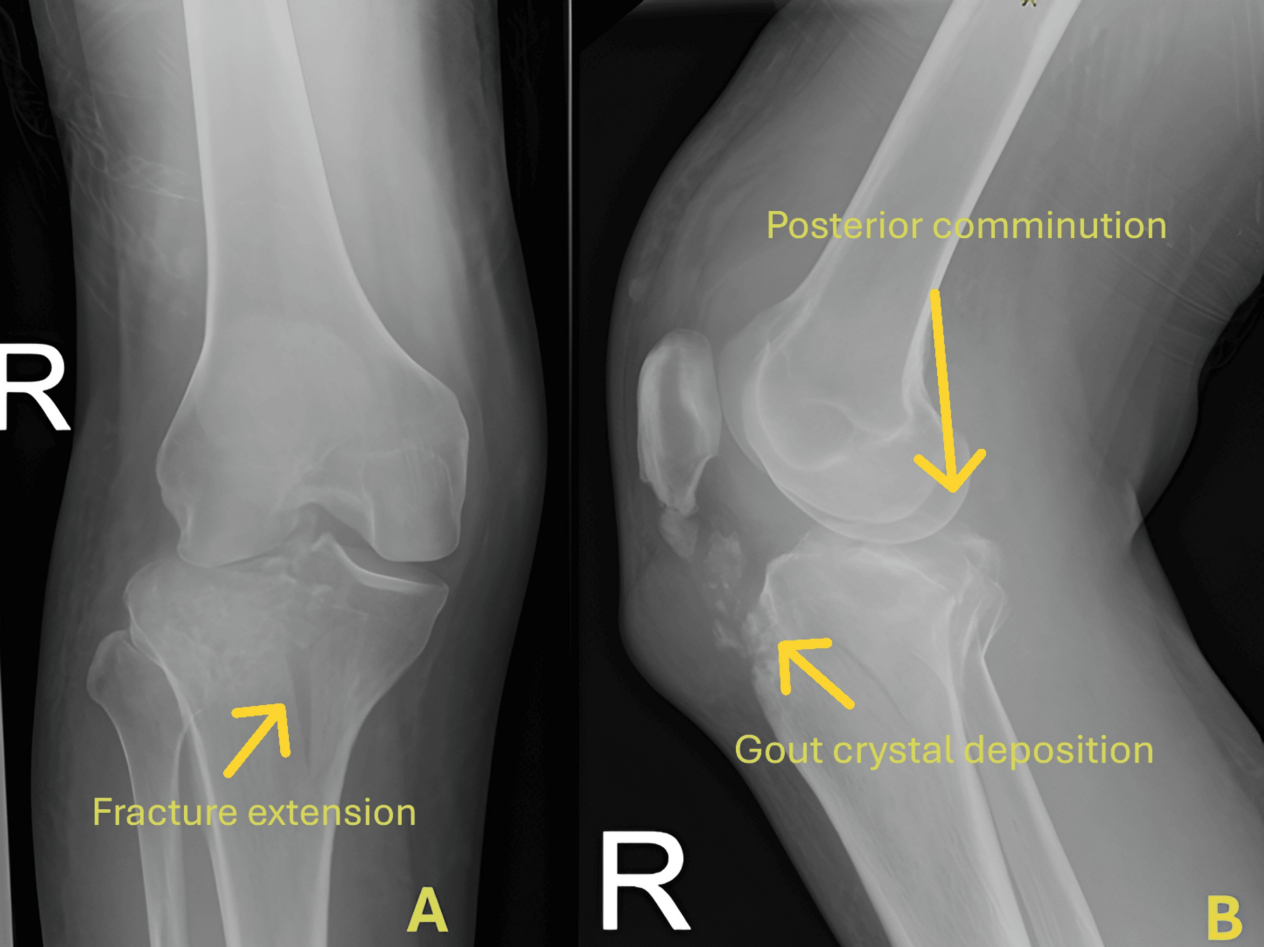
Radiograph showing (A) lateral tibial plateau depression with metaphyseal-diaphyseal extension and (B) posterior fragments. Calcification over the patella tendon is due to chronic gouty arthritis
